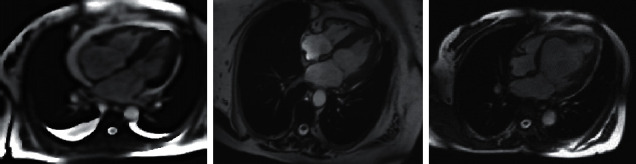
CNN denoised MRI images.
